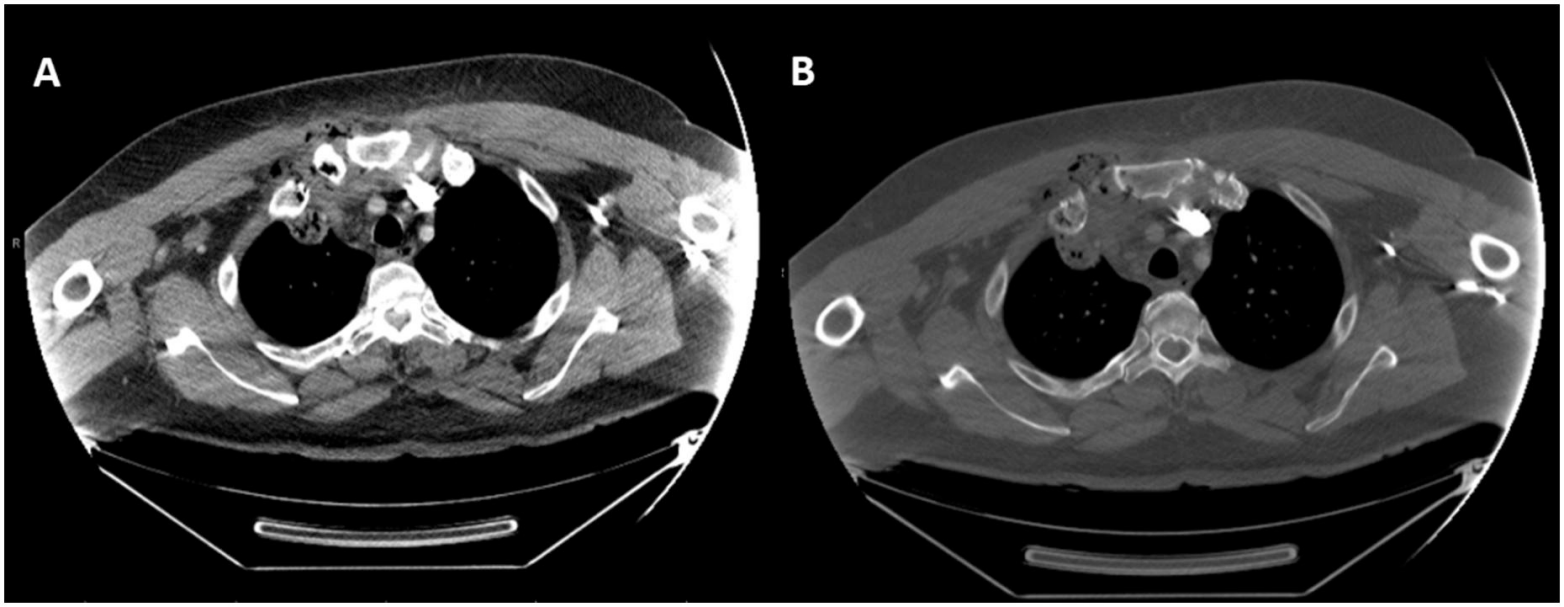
Contrast-enhanced CT of the chest in soft tissue (A) and bone (B) windows demonstrating intraosseous gas and cortical erosions of the right first rib, right clavicular head, and adjacent manubrium, highly concerning for emphysematous osteomyelitis. Additionally, emphysematous septic arthritis of the right sternoclavicular joint is evident by swelling of the joint and the intraarticular gas. Associated with stranding and emphysema affecting the surrounding soft tissues, the right pectoralis major, and the anterior upper mediastinum, concerning for necrotizing fasciitis.
